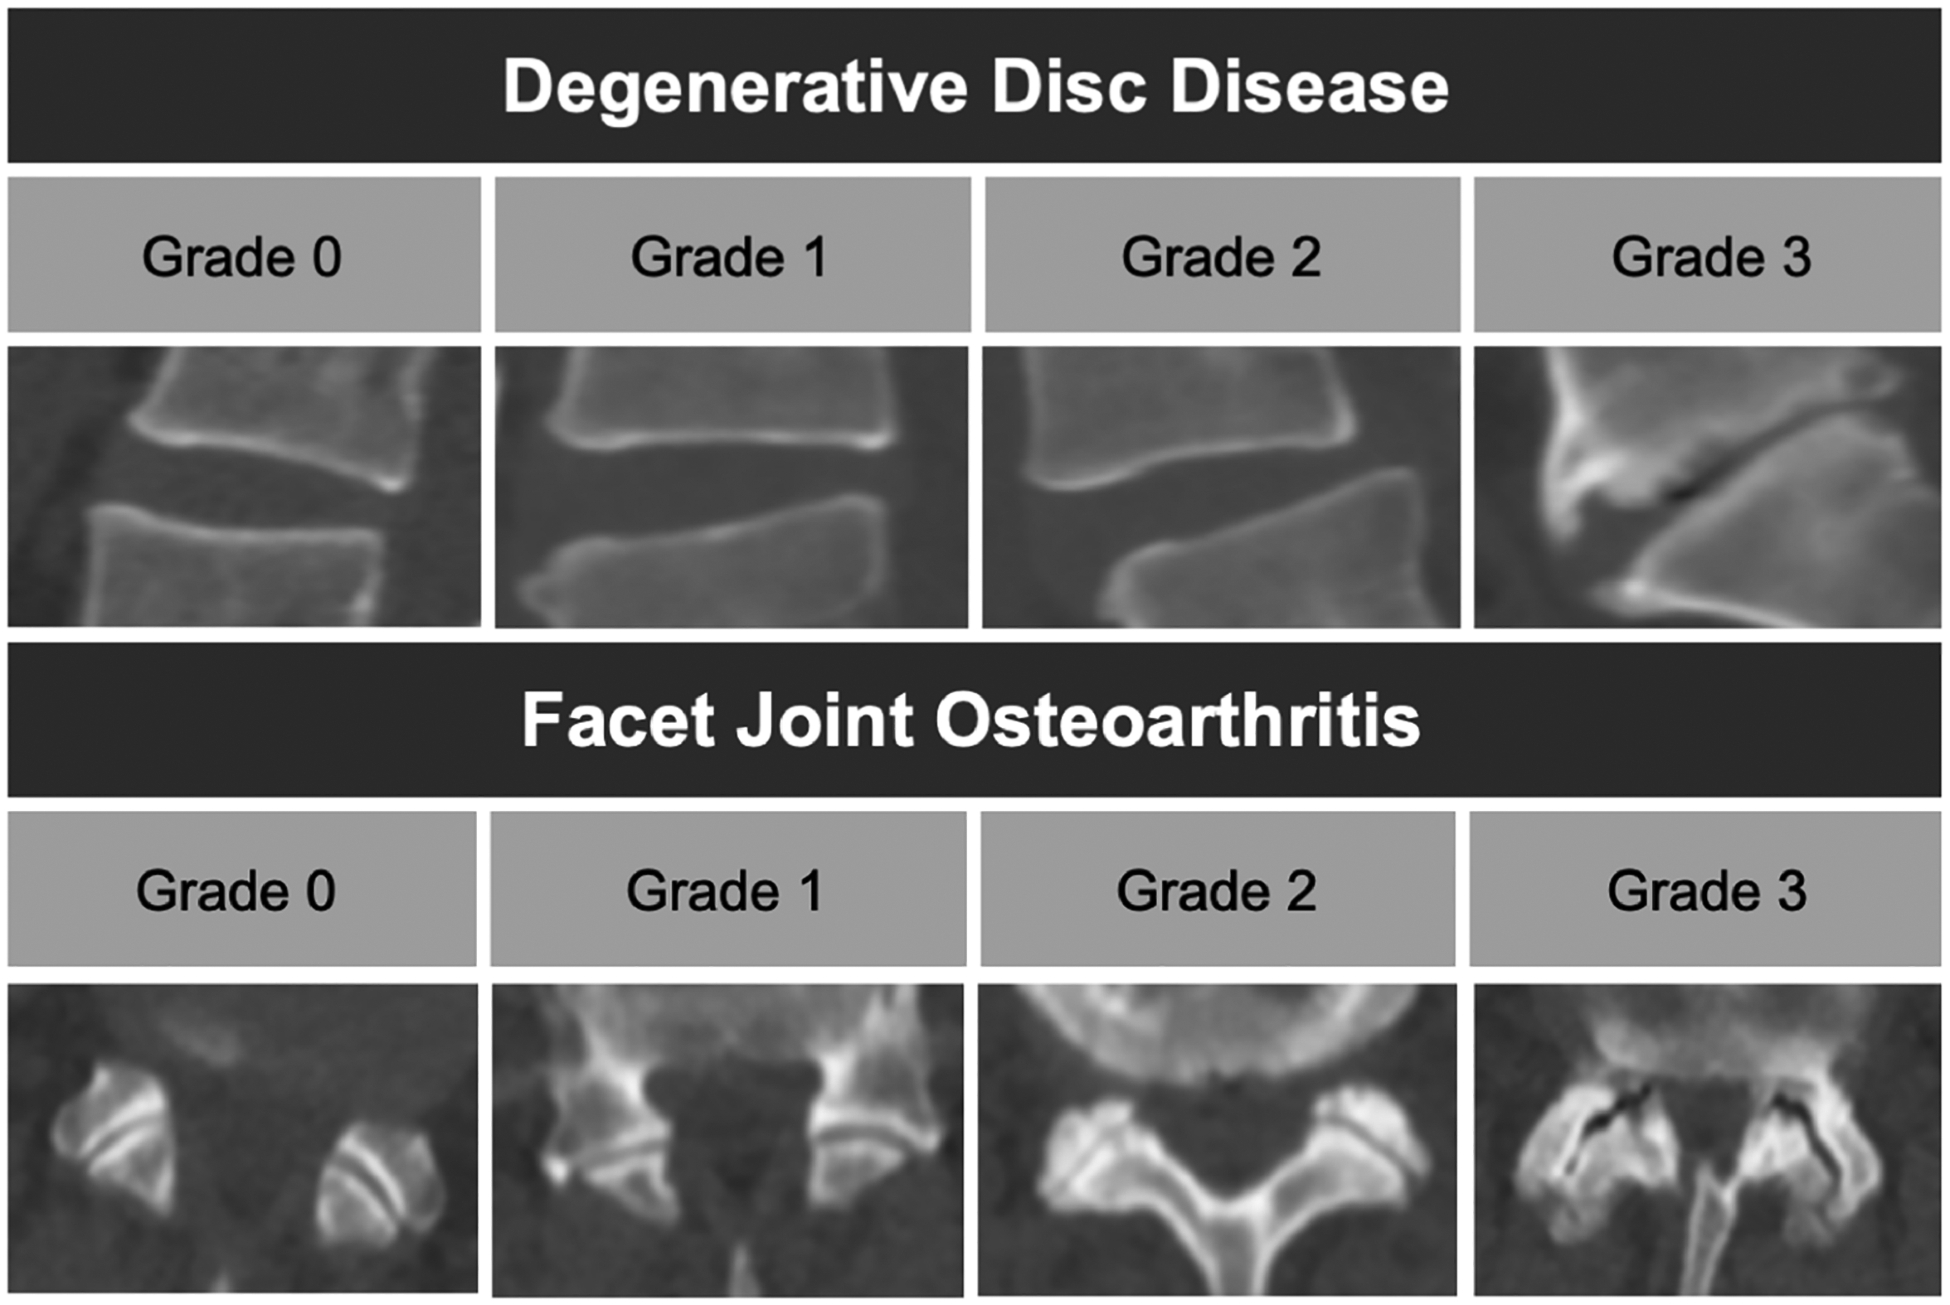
Representative CT images of grades 0–3 degenerative disc disease and facet joint osteoarthritis. Degenerative disc disease was assessed in the sagittal plane on a scale of 0 to 3, where 0 = normal, disc height greater than the cephalad disc (except for the L5–S1 disc); 1 = disc height equal to the cephalad disc if it is normal, with no to slight disc bulging; 2 = disc narrower than the cephalad disc if it is normal (generally associated with disc bulging anteriorly and/or posteriorly); and 3 = severe narrowing, with end plates almost in or in contact. Facet joint OA was quantified in the axial plane on a scale of 0 to 3, where 0 = normal; 1 = mild, with narrowing of the joint space (<2 mm) and small osteophytes and/or mild hypertrophy of the articular process; 2 = moderate, with narrowing of the joint space (<1 mm) and moderate osteophytes, moderate hypertrophy of the articular process, or mild subarticular bone erosions; and 3 = severe, with profound narrowing of the joint space and large osteophytes, severe hypertrophy of the articular process, severe subarticular bone erosions, or subchondral cysts.
